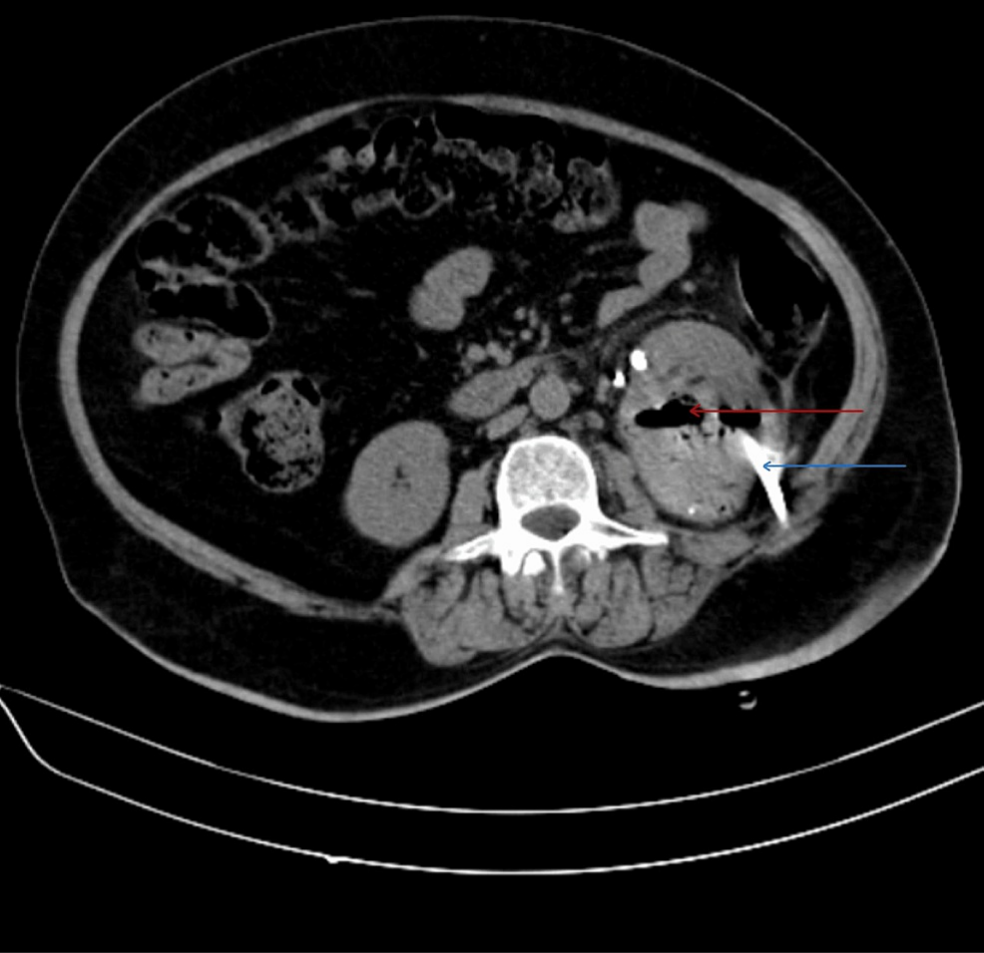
Non-contrast computed tomography of the abdomen showing the left kidney appearing enlarged with air foci and fluid attenuation collection within it (red arrow) with percutaneous nephrostomy catheter seen in-situ (blue arrow).
